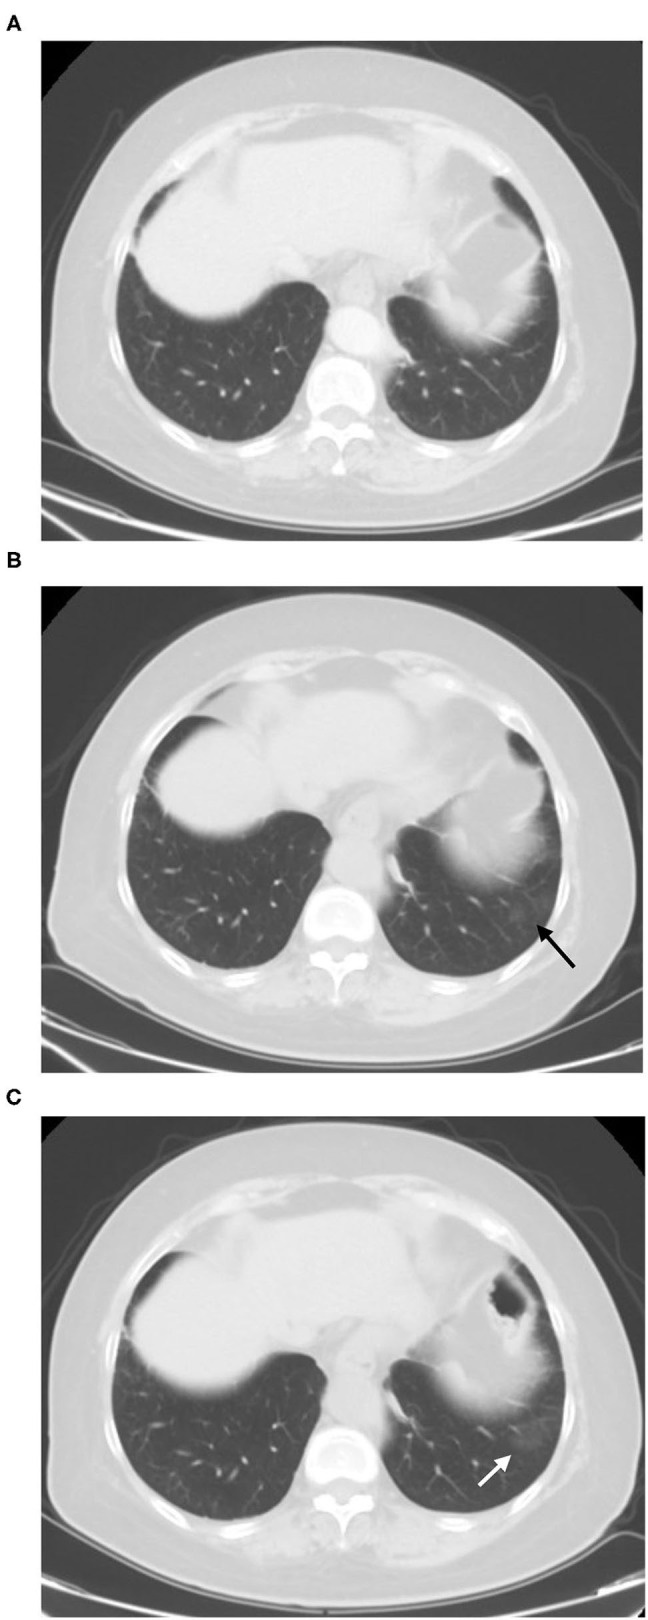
Sixty-seven-year-old female with post-target therapy of lung cancer history. Screening CT scan (B) showed a pure GGO lesion can be newly seen in the left lower lobe (arrow) comparison to the prior CT scan which performed at 2 months ago (A). The short-term follow-up CT showed increases in the size of GGO in the lungs [(C), white arrow]. The RT-PCR results of the patient was negative.
